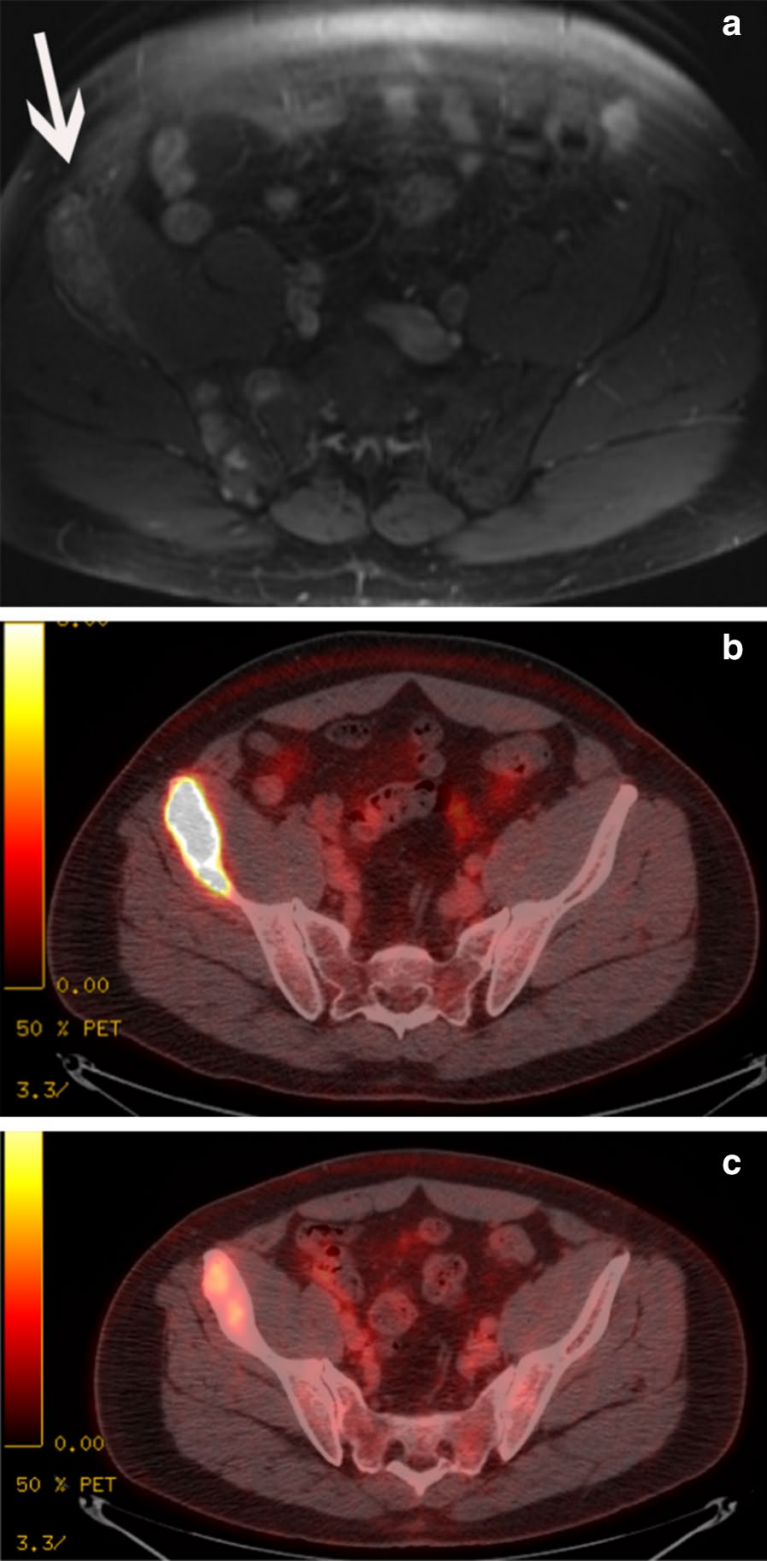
Imaging studies for 45-year-old patient (“Case 1”) with pseudomyogenic hemangioendothelioma. a Baseline fat saturated T1-weighted axial magnetic resonance image with intravenous contrast enhancement of the pelvis demonstrates multiple enhancing tumor foci in the right iliac bone (*white arrow*). b Positron emission tomography-computed tomography (PET-CT) fusion image through the pelvis demonstrates diffuse metabolic activity in the right anterior iliac bone (disease in the right posterior iliac bone at the level of the sacroiliac joint was also present but is not shown). This image was obtained 1 month before the patient began gemcitabine/docetaxel. c PET-CT fusion image through the pelvis after three cycles of gemcitabine/docetaxel demonstrates a significant metabolic response
